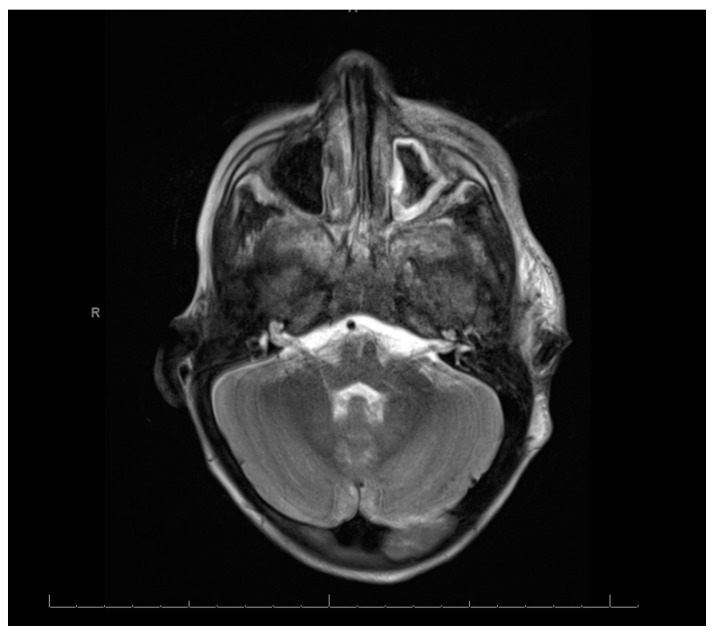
Brain and neck computed tomography with contrast (cross-sectional view).
